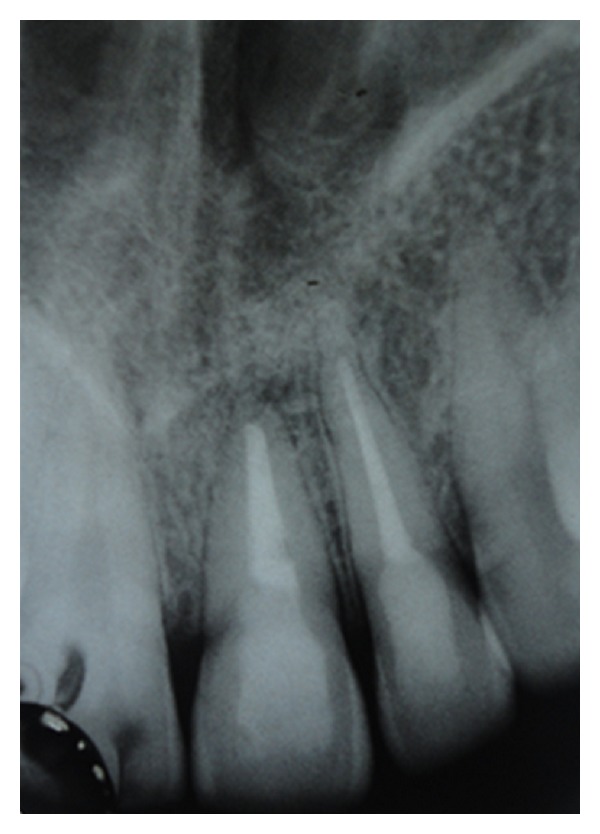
Follow-up radiograph after 12 months.
